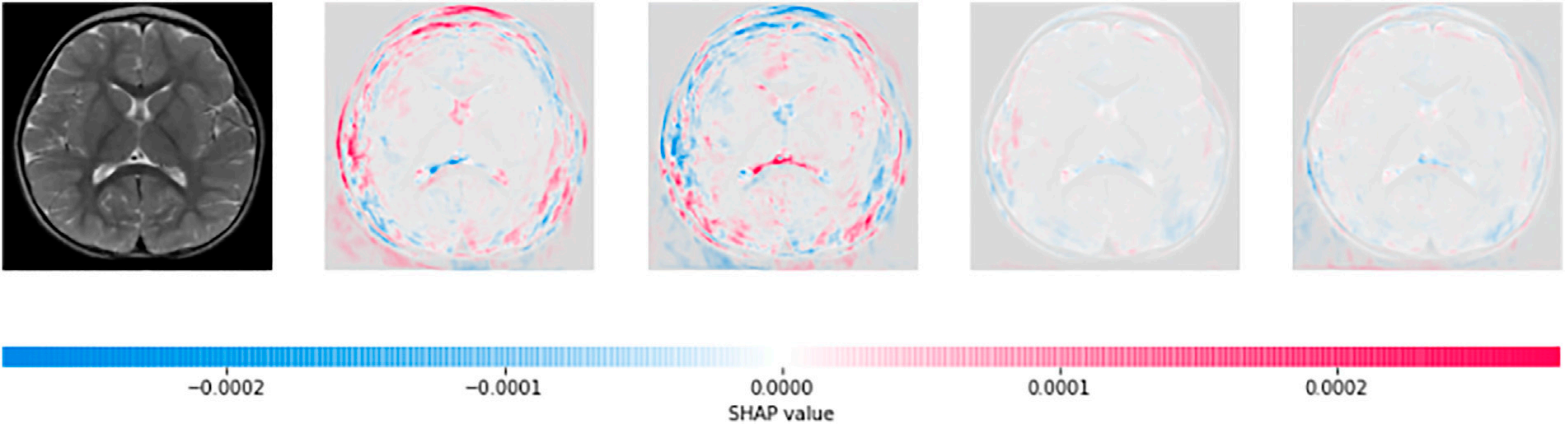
On the basis of Shapley values, we can say that the MRI image is normal.
